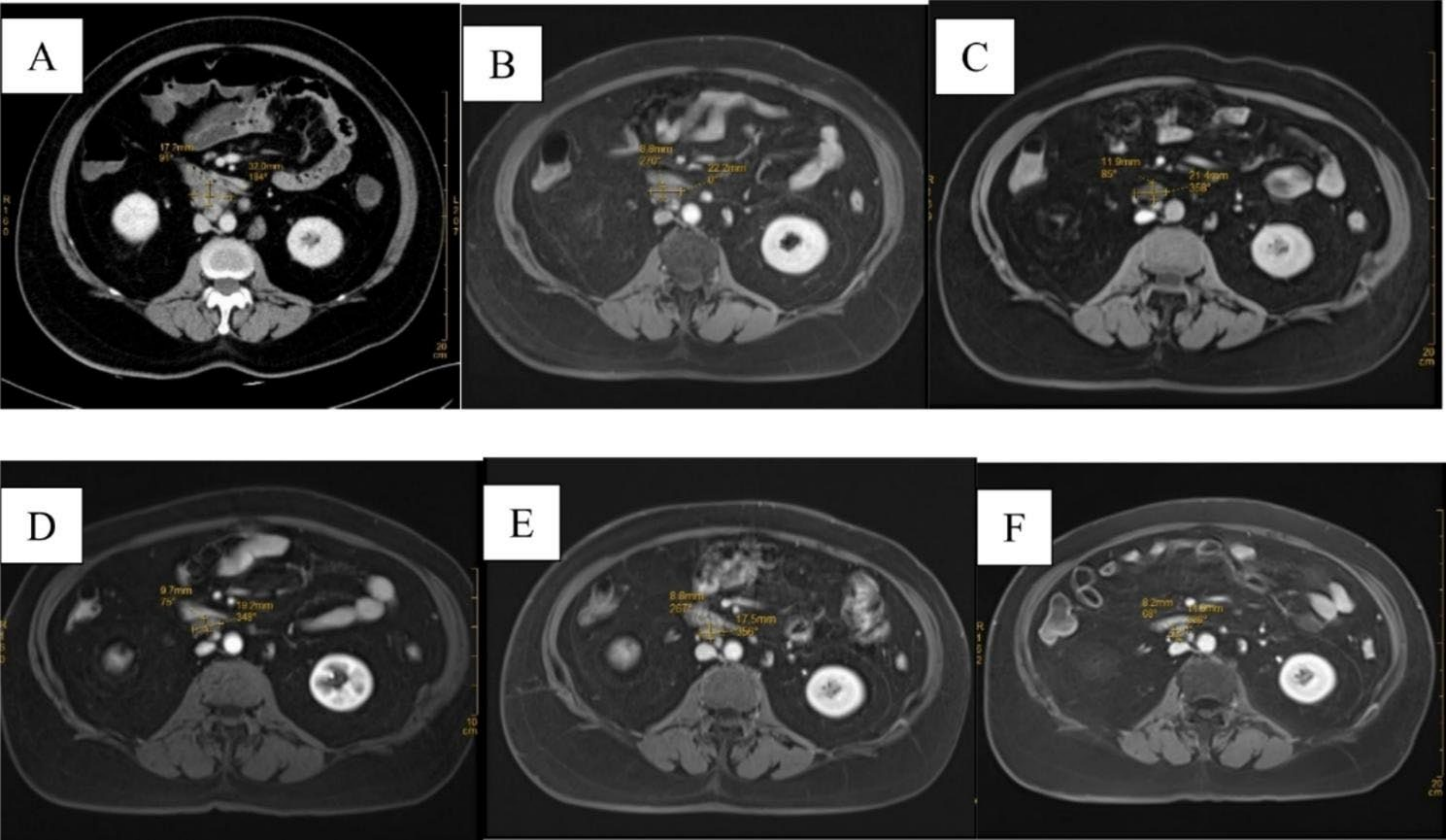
Serial Imaging of abdomen; A: CT abdomen showed the size of the largest retroperitoneal lymph node was 17.2 × 32.0 mm on July 2018 (Before treatment); B: MRI abdomen showed the size of the largest retroperitoneal lymph node was 8.2 × 22.2 mm on November 2018 (After 4 months of Doxycycline and Hydroxychloroquine); C: MRI abdomen showed the size of the largest retroperitoneal lymph node was 11.9 × 21.4 mm on July 2019 (After 6 months of trimethoprim/sulfamethoxazole); D: MRI abdomen showed the size of the largest retroperitoneal lymph node was 9.7 × 19.2 mm on January 2020 (After 12 months of trimethoprim/sulfamethoxazole); E: MRI abdomen showed the size of the largest retroperitoneal lymph node was 8.8 × 17.5 mm on July 2021(After 18 months of Doxycycline and Hydroxychloroquine); F: MRI abdomen showed the size of the largest retroperitoneal lymph node was 8.2 × 14.6 mm on May 2022 (After 28 months of Doxycycline and Hydroxychloroquine)
